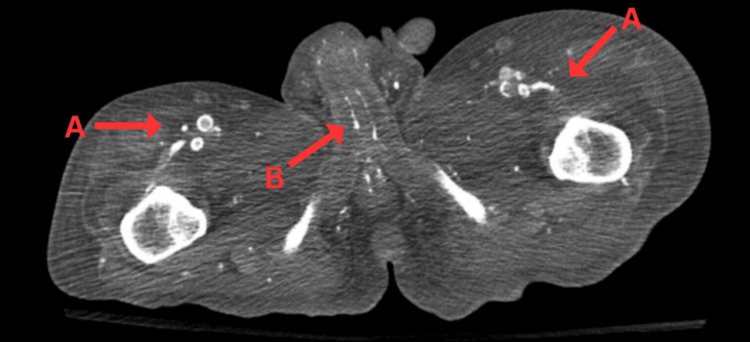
Axial view of abdominal/pelvic CT scan. The above figure demonstrates (A) diffuse calcification of the femoral artery and branches and (B) calcification of the penile terminal branches of the internal pudendal artery.
